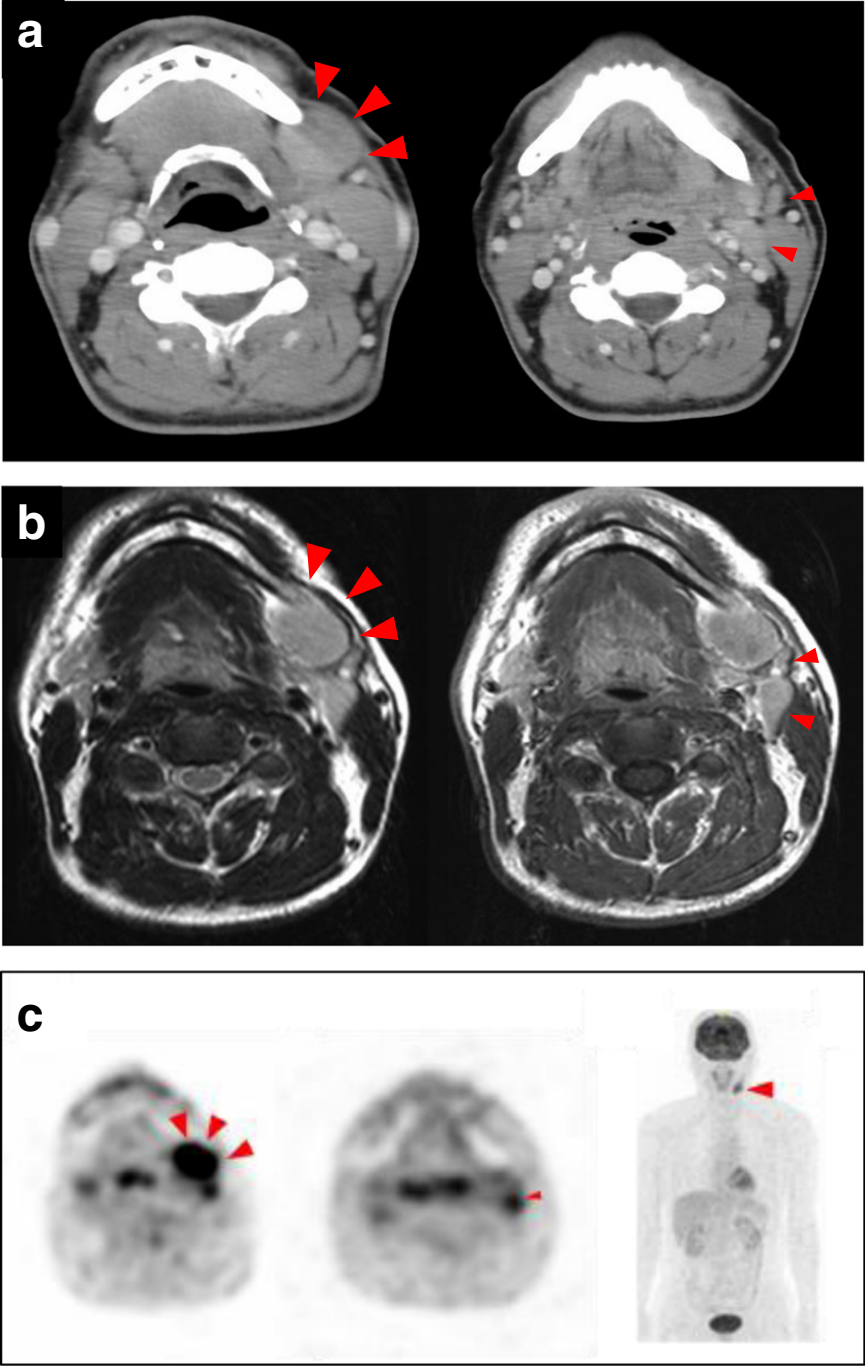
CT scan (a) and Contrast-enhanced T1-weighted MRI (b) revealed an oval swelling (*Large arrowhead*) in the left submandibular region associated with enlarged submental lymph nodes (*Small arrowhead*) and the superior internal jugular area. A PET (b) revealed abnormal accumulation of FDG in the left submandibular (*Large arrowhead*) and left upper internal jugular regions (*Small arrowhead*). No clear abnormal accumulation was noted in any area other than the neck region
